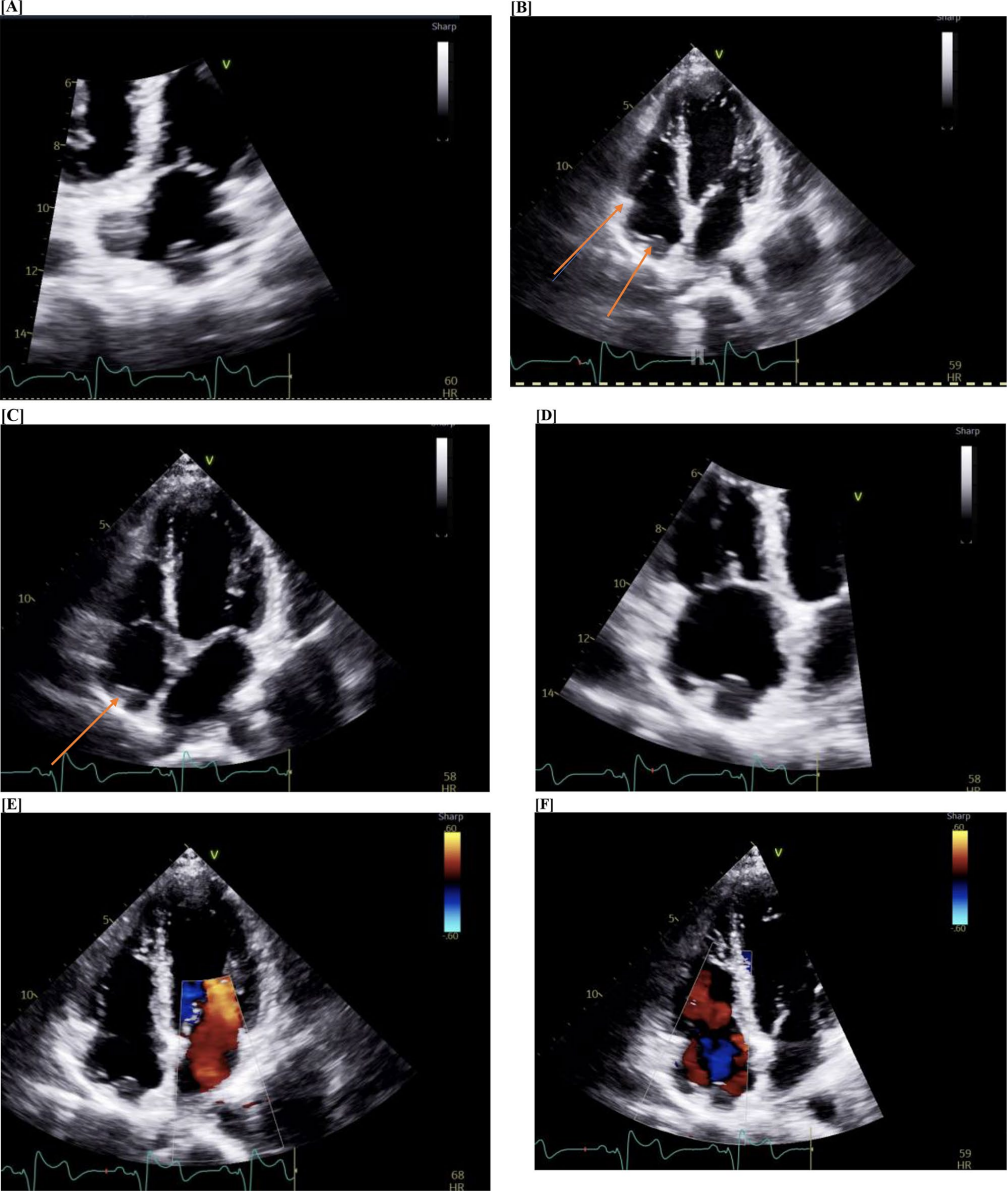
Transthoracic Echo Detecting Mass (A to F)
